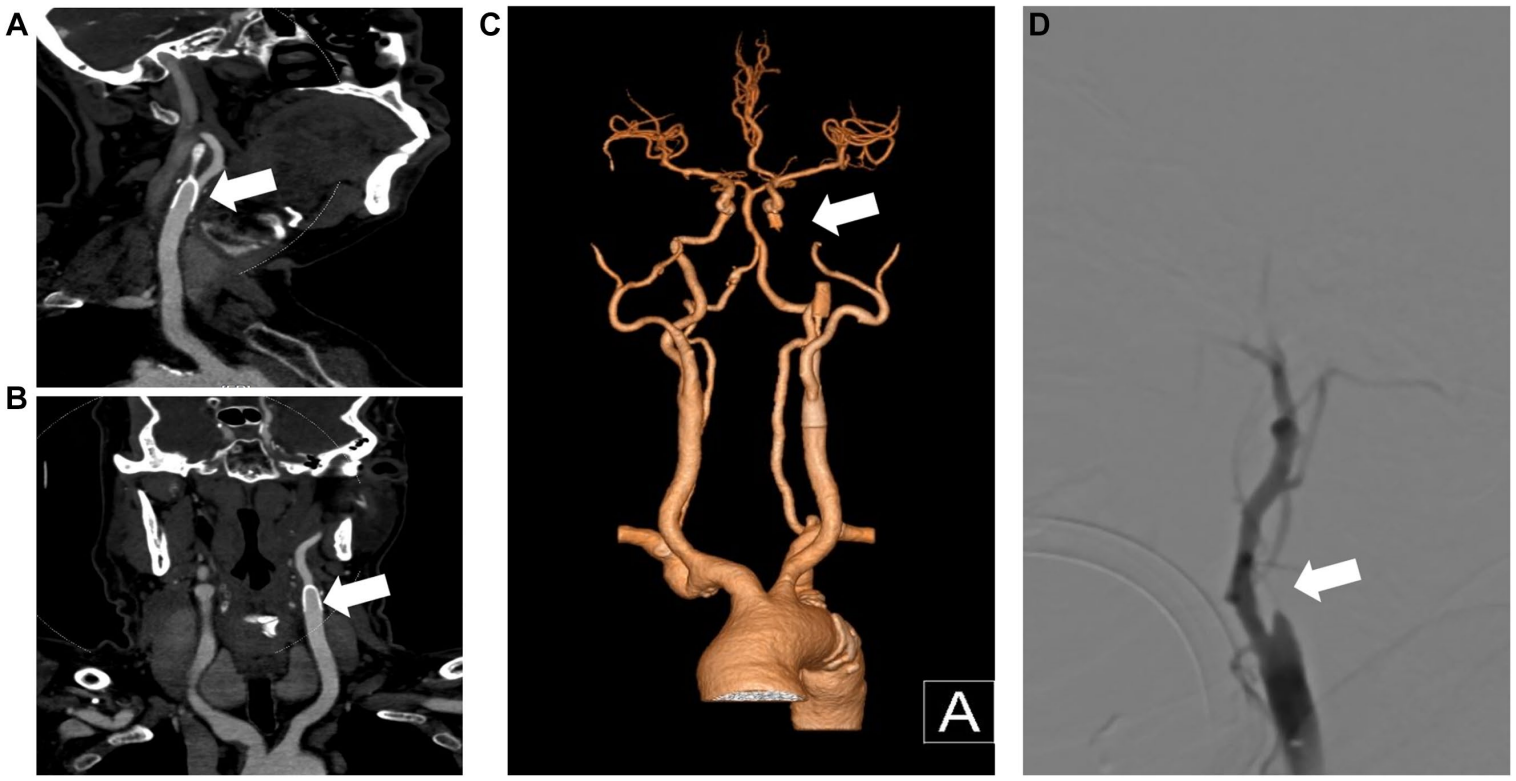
CTA and DSA show carotid stent thrombosis. The sagittal plane (A), coronal plane (B) and 3D reconstruction (C) of the CTA show stent thrombosis. DSA confirmed left internal carotid artery thrombosis and stent occlusion (D). CTA, computed tomography angiography; DSA, digital subtraction angiography; 3D, three-dimensional.
